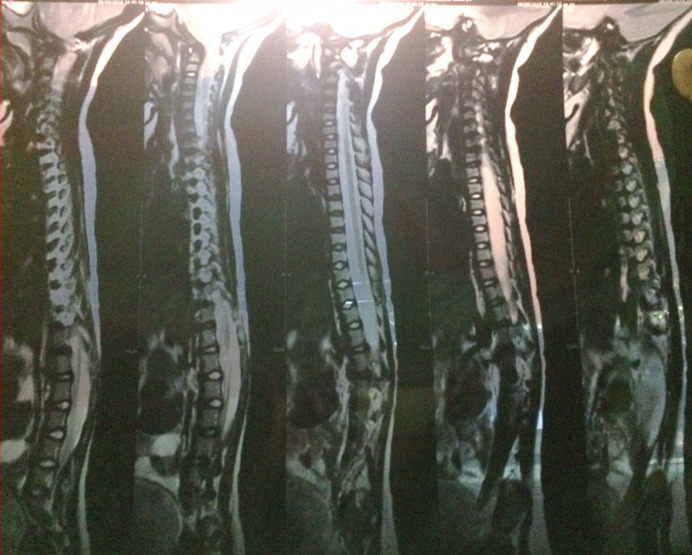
magnetic resonance imaging (MRI) scan screening of whole spine
